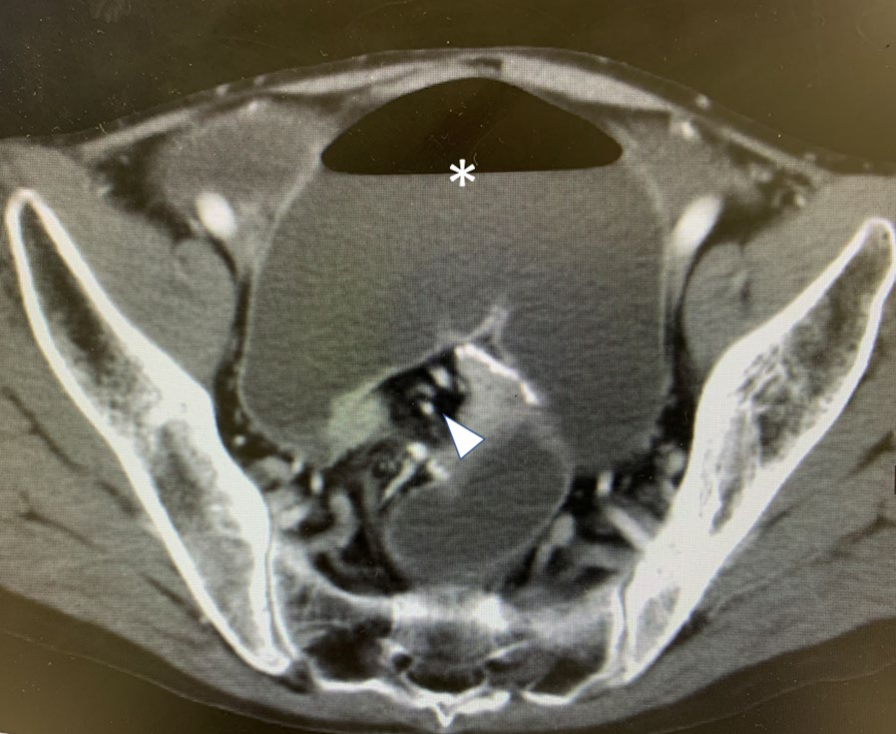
Enhanced multidetector computed tomography demonstrates a whirl sign at the upper corner of the pouch. White up-pointing triangle: superior mesenteric artery and vein, *: dilated oral ileum
